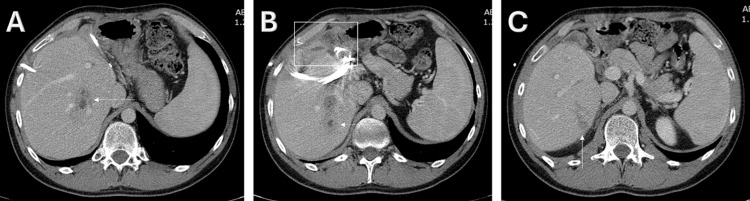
Follow-up CECT scan of the abdomen, after 12 days of antibiotics A: slight decrease in the size of the abscess at the junction of segments VII and VIII (arrow); B: slight decrease in the size of the abscess at segment VII (arrow), as well as in the subcapsular abscesses at segments IV-B and VIII (square); C: decrease in the extension of the ill-defined hypodense lesions previously seen in segments V and VI (arrow). CECT: contrast-enhanced computed tomography
